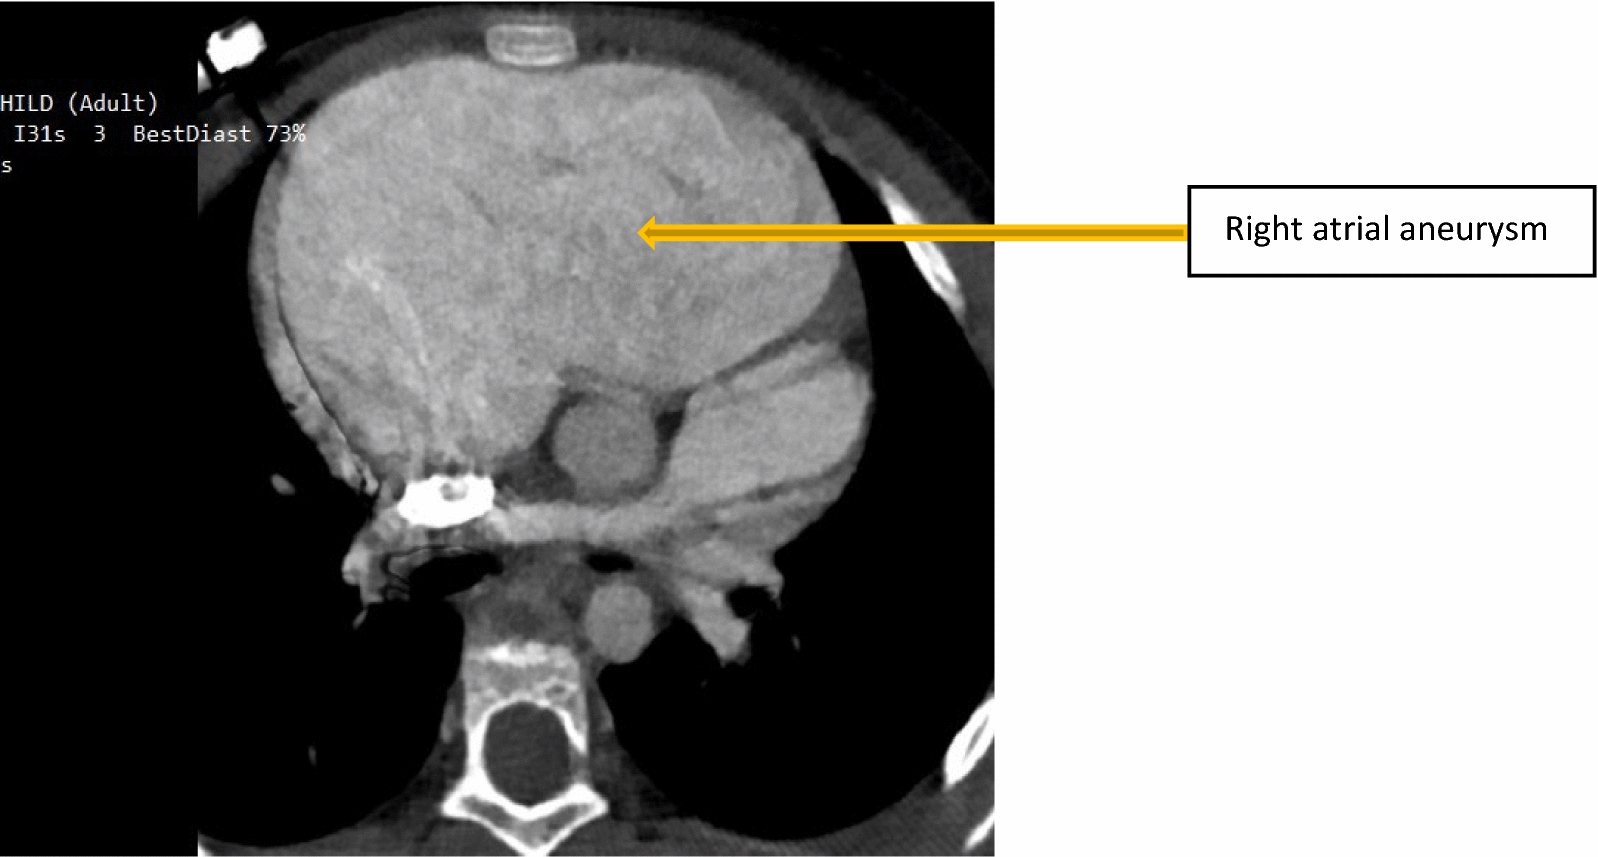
Contrast cardiac computed tomography scan showing large right atrial aneurysm
